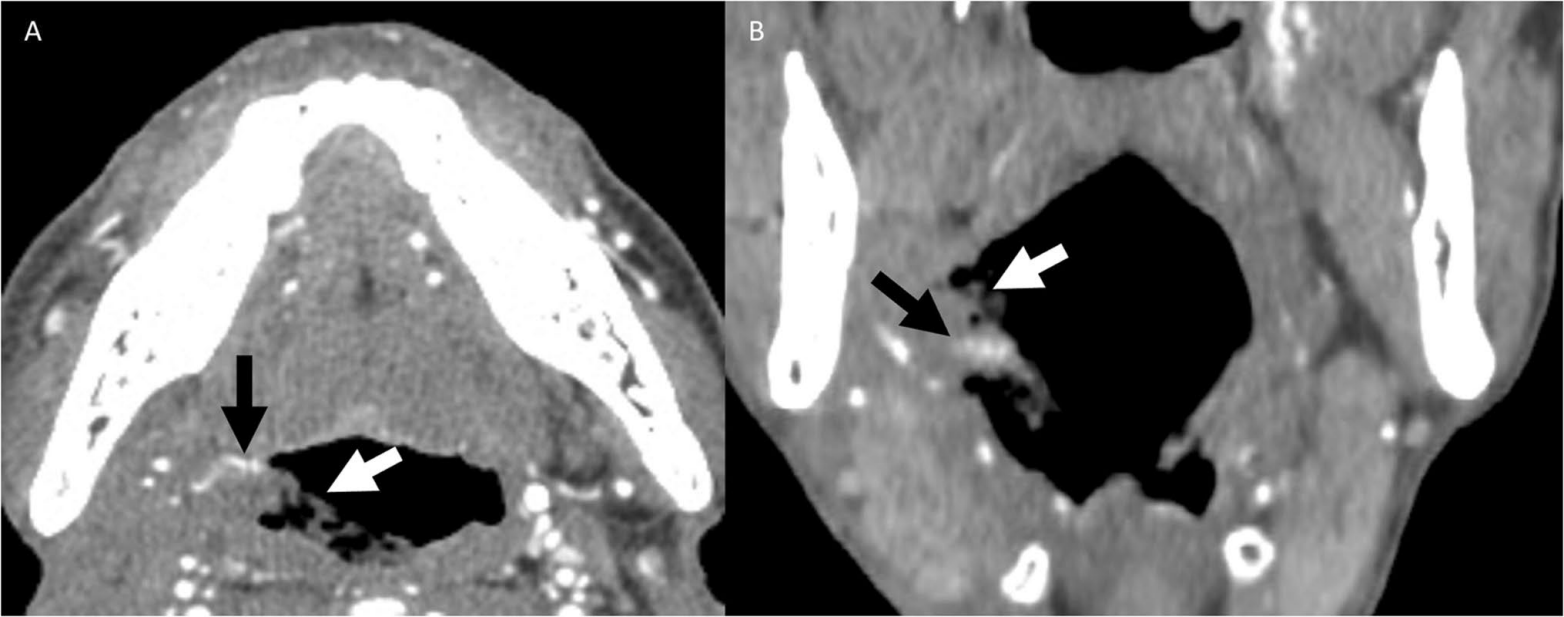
Patient 1. Axial (A) and coronal (B) postcontrast CTA images of the neck at the level of the base of tongue demonstrate linear high-density contrast material (black arrow) surrounded by low-density foamy debris (white arrow) in the right oropharyngeal lumen, compatible with active hemorrhage
